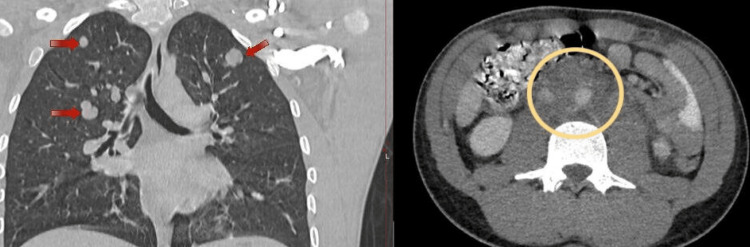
CT scan of the abdomen and chest for cancer staging demonstrating bilateral pulmonary metastasis (left - red arrow) and multiple retroperitoneal lymph nodal involvement (right - encircled in yellow) CT scan of the abdomen and chest for cancer staging demonstrating bilateral pulmonary metastasis (left - red arrow) and multiple retroperitoneal lymph nodal involvement (right - encircled in yellow)
